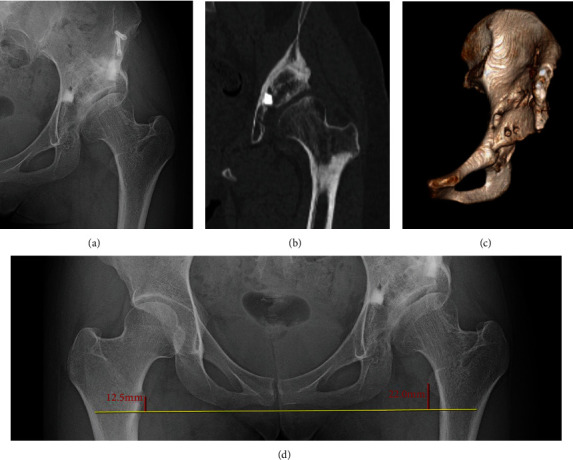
Anteroposterior (AP) radiograph and CT images taken three years after surgery showing the following: (a) AP radiograph of the left hip joint, (b) CT coronal view, and (c) 3D-CT frontal view. Although the superior acetabular coverage was still insufficient, the compatibility of the left hip joint was improved (d). When measured, the postsurgery leg length discrepancy was 9.5 mm.
